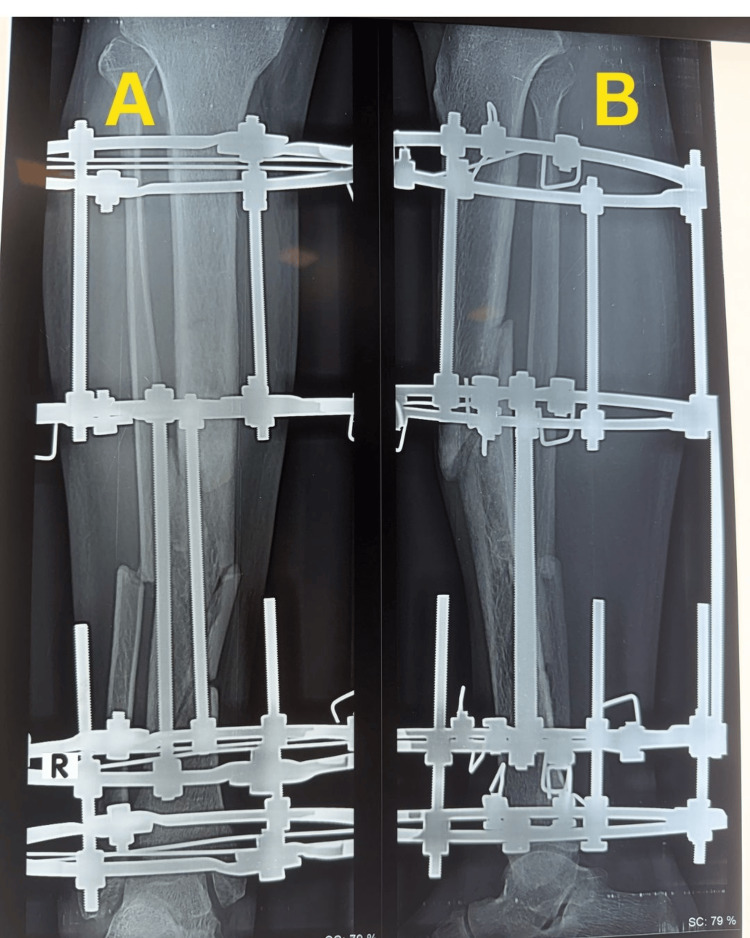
Follow-up radiographs showing progressive fracture healing Follow-up radiographs obtained on 18 February 2026 showing progressive callus formation and ongoing fracture healing: anteroposterior view (A) and lateral view (B).
